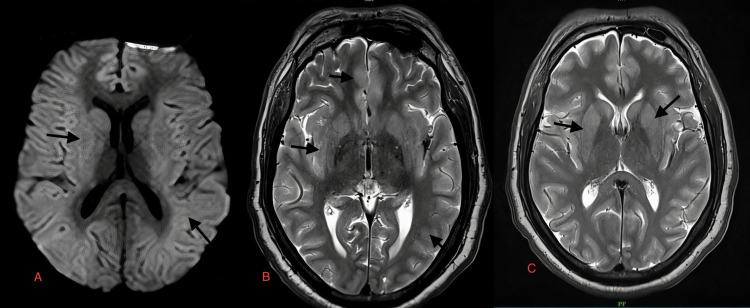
Case 4: Diffuse areas of diffusion restriction in DWI image are seen in both cerebral hemispheres including gray white matter, deep white matter, and basal ganglia region with sparing of the thalami and frontal white matter (A) in a 17-year-old male with dengue encephalitis. Corresponding hyperintensities are seen on T2W images (black arrows in B and C). DWI: diffusion-weighted image; T2W: T2-weighted image
